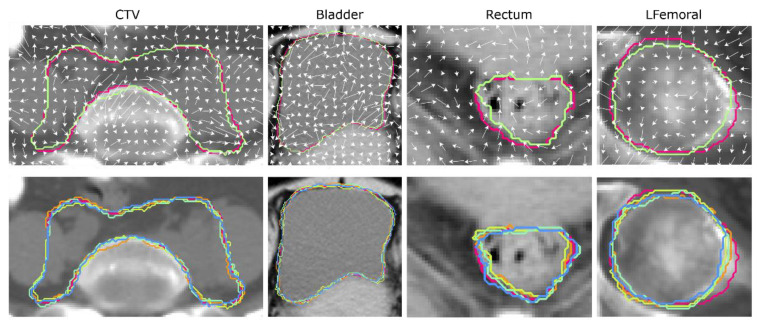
The first row shows random displacement fields (white arrows) in CTV, bladder, rectum, and LFemoral and two randomized contours for the four ROIs (red: original contour, light green: randomized contour) overlayed with the CT image. The second row shows four different randomized contours with different colored lines in addition to the original contour (red line).
